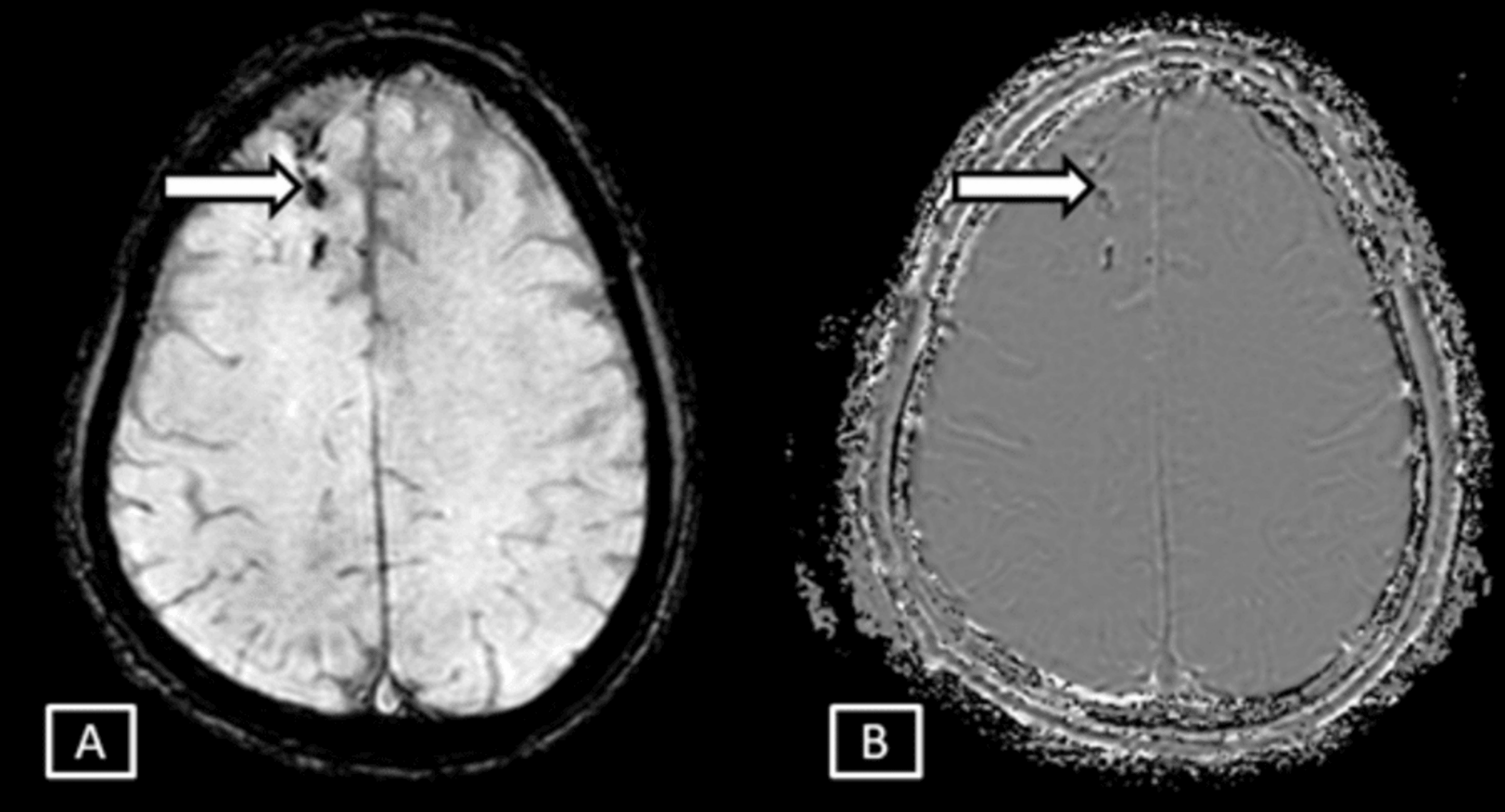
Case 1 MRI axial brain magnitude (A) and phase (B) SWI sequences. Images show corresponding hypointensites in the phase and the magnitude sequences represented by the white arrows. SWI: Susceptibility Weighted Imaging
